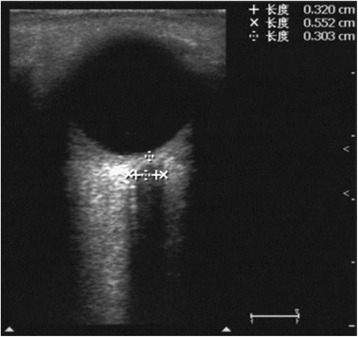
Transbulbar sonography of the optic nerve and the optic nerve sheath. OND and ONSD were measured 3 mm behind the globe using an electronic caliper along the axis. The interval between “+” marks was OND, and the interval between “x” marks was ONSD
